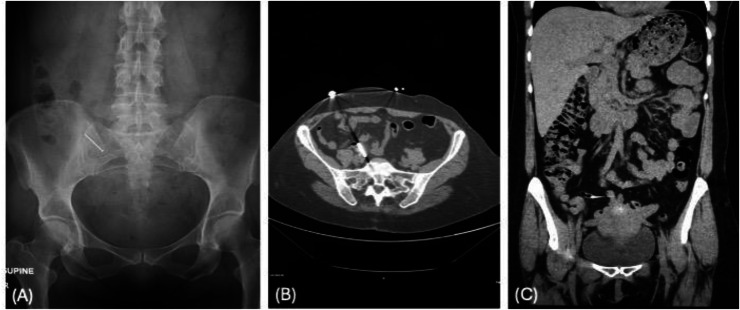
(A) Abdominal X-ray obtained three weeks after ingestion, showing the object persisting in the right lower quadrant. (B) Axial CT image showing the object within the appendix. (C) Coronal CT image confirming intra-appendiceal location without radiologic signs of inflammation or perforation.
